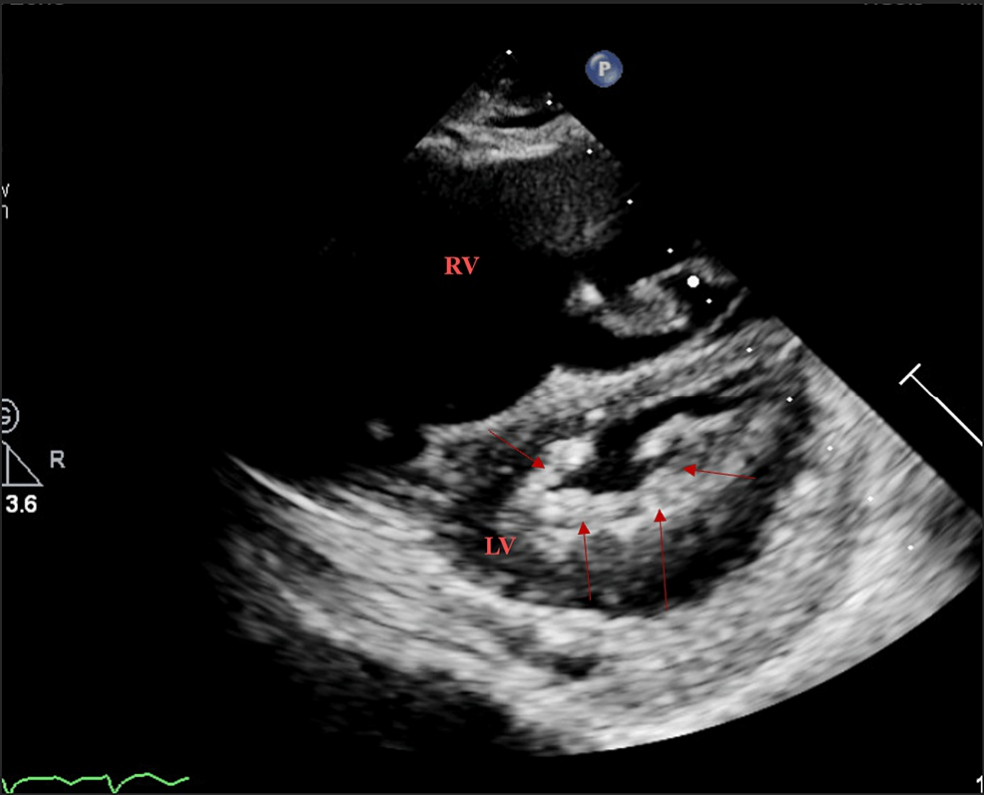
Parasternal short-axis with restricted mitral orifice and diffuse thickening surrounding the orifice (arrows) RV: right ventricle, LV: left ventricle
